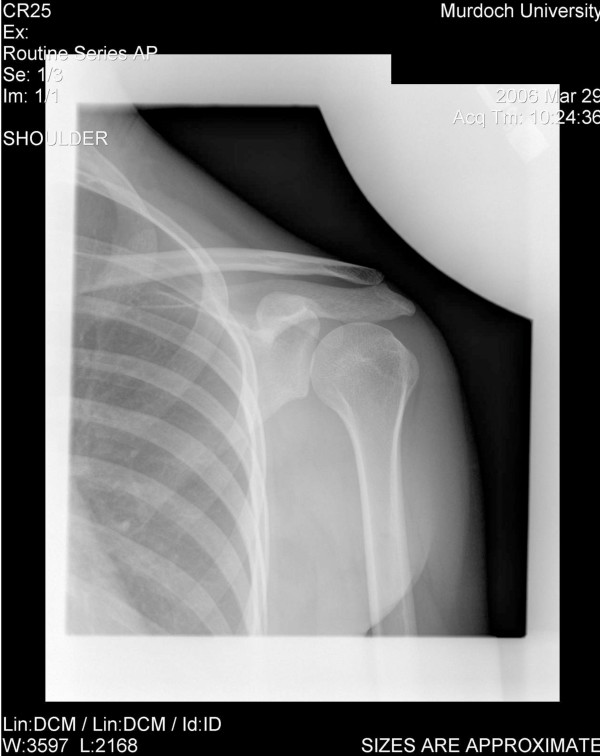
see attached jpeg file named "XRay 1".
